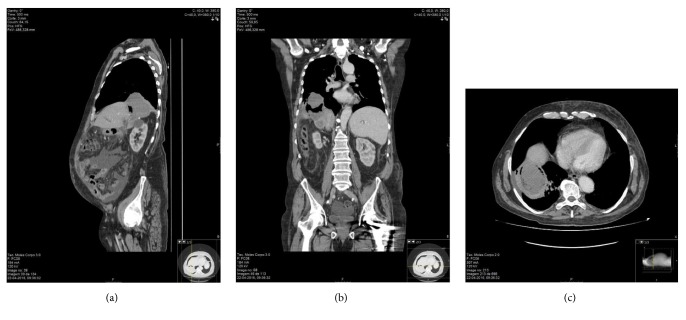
CT-Scan performed in April 2016: (a) sagittal plane, (b) coronal plane, and (c) transverse plane.
